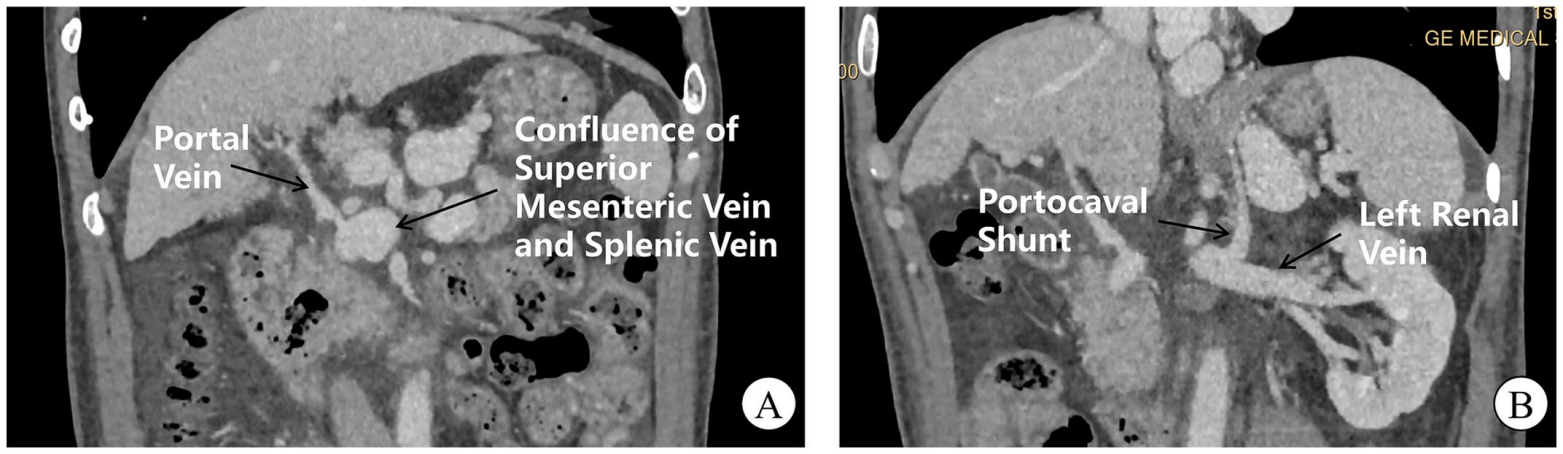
CT venography of portal and systemic circulation. (A) Hepatic arteriography reveals an attenuated main portal vein with a prominent aberrant shunt vessel (arrow) draining directly into the inferior vena cava at the hepatic hilum. Note dilation and tortuosity of the superior mesenteric and splenic veins converging into the shunt. (B) A small-caliber left subphrenic vessel (arrowhead) drains into the left renal vein.
